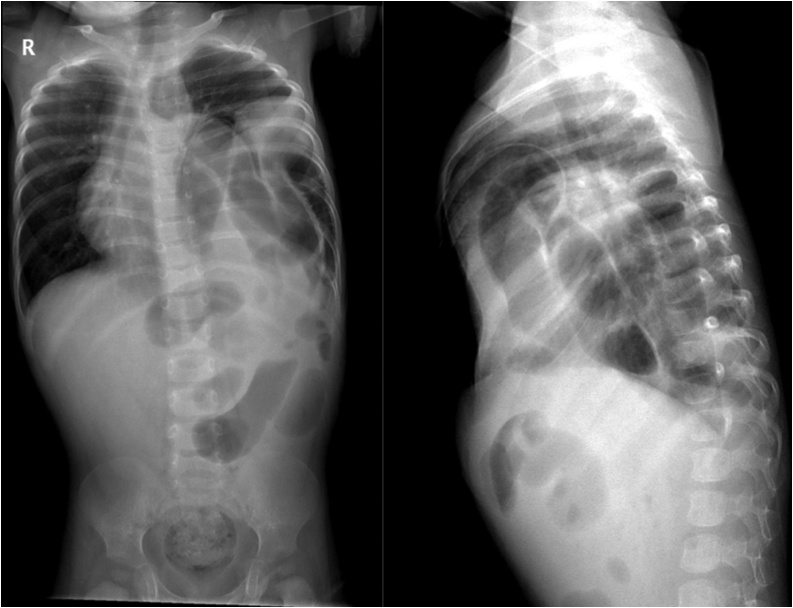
Anteroposterior and lateral chest x-ray showing strangulation CDH.
